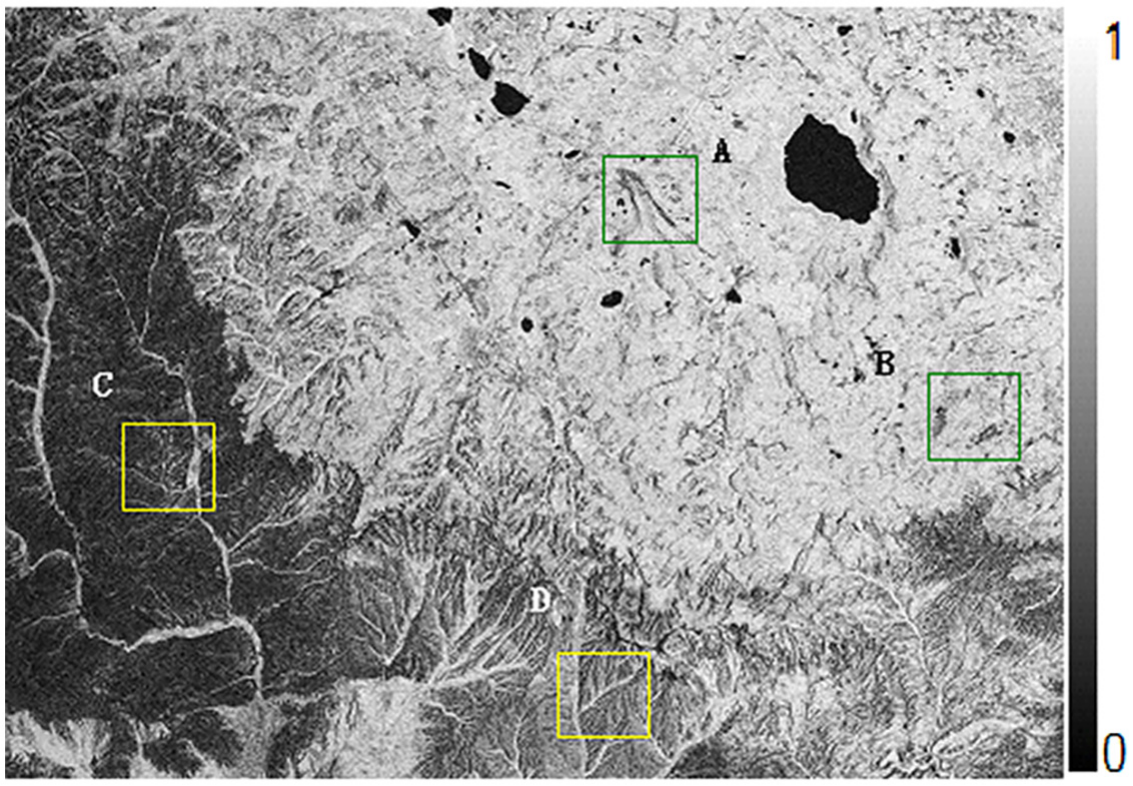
Coherence map from level 1 data without consistent doppler parameters.
